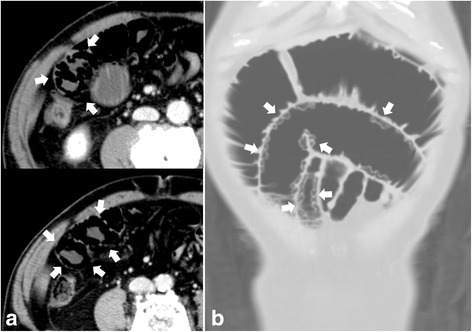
Computed tomography (CT) scan findings. Abdominal CT scans revealed dilatation of the small bowel from the duodenal third portion to the jejunum. Axial sections revealed dilated loops with a ring-like appearance due to intramural gas (a). Coronal section revealed multiple gas-filled cysts along the wall of the dilated jejunum (b). Arrows indicate pathognomonic intramural gas
